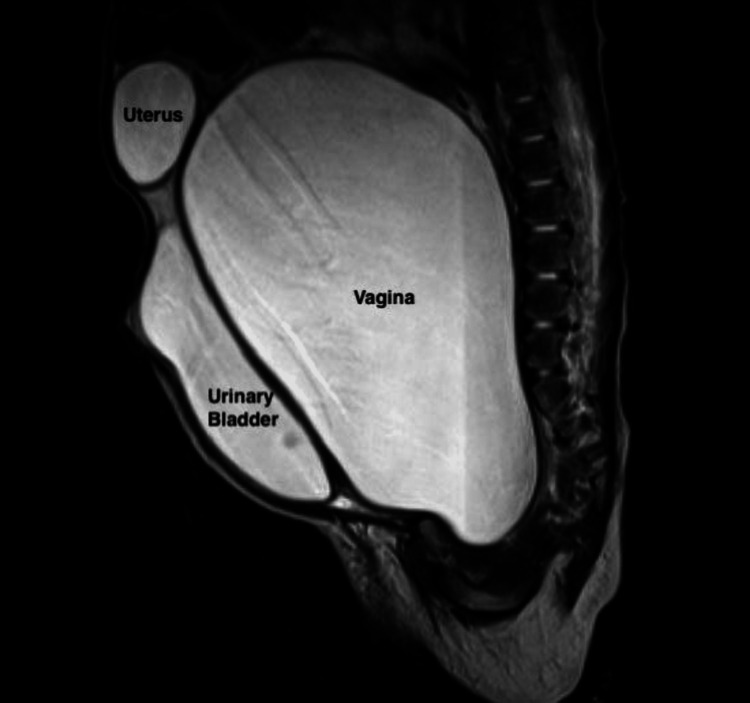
MRI in the sagittal plane shows the presence of a distended vagina containing fluid. The uterus is displaced superiorly, and the urinary bladder is compressed and pushed anteriorly.
